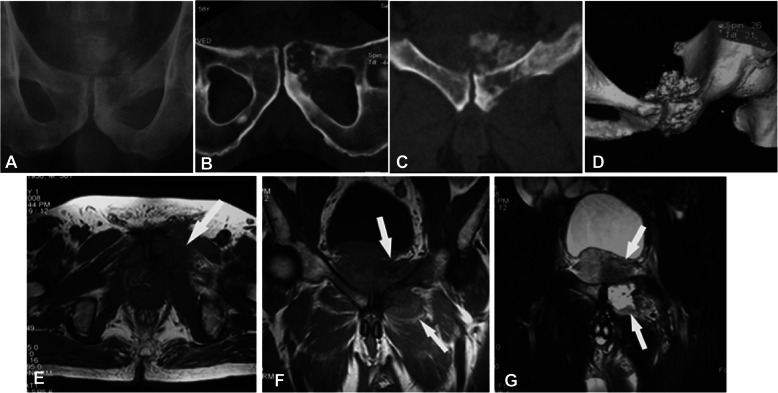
A 58-year-old man had left leg thigh pain for 3 months and was diagnosed with pubic dedifferentiated chondrosarcoma. a Plain radiograph demonstrated bone destruction at the pubic symphysis with ill-defined margins and irregular high-density lesion. b, c Computed tomography (CT) reconstruction displayed the pubic destruction, punctiform calcification and ossification, and a soft tissue mass. d Three-dimensional CT reconstruction revealed pubic destruction and a pelvic mass. e-g Magnetic resonance imaging showed unevenly long T1WI (e and f) and long T2WI (g) signal, with ill-defined margins, calcification, and ossification. The expansile destruction and calcification were typical for chondrosarcoma
